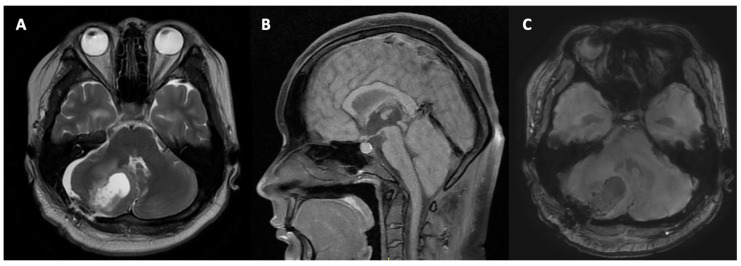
Postoperative MRI (day 1). Axial T2WI (A), sagittal T1WI (B), and axial 3D eSWAN (C) obtained 1 day after surgery demonstrated adequate decompression of the posterior fossa with restoration of fourth ventricle patency. No residual cystic lesion was identified. Early regression of ventricular dilatation was noted, without radiological signs of acute postoperative complications.
